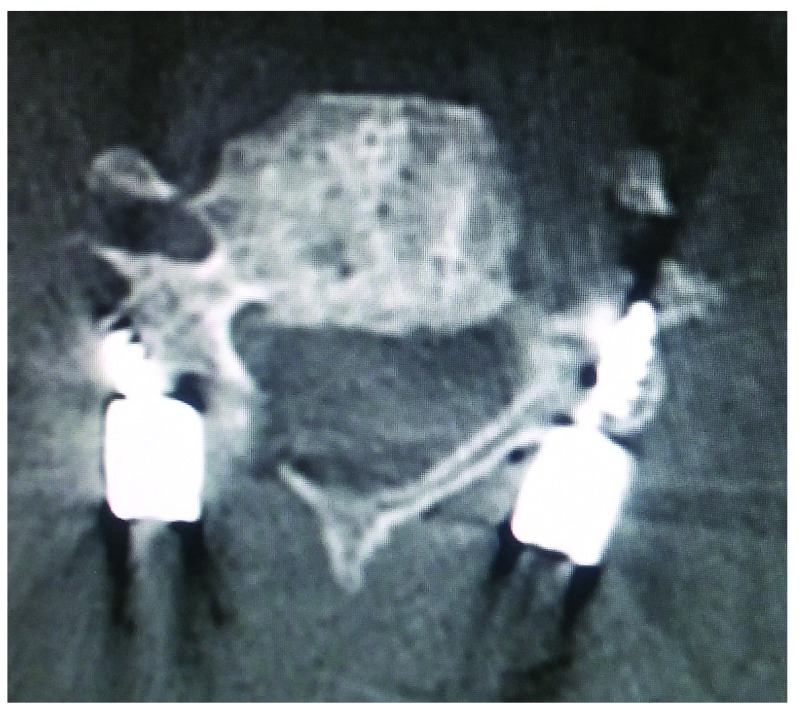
CT spine showing projection of screw through lateral mass of C3.
